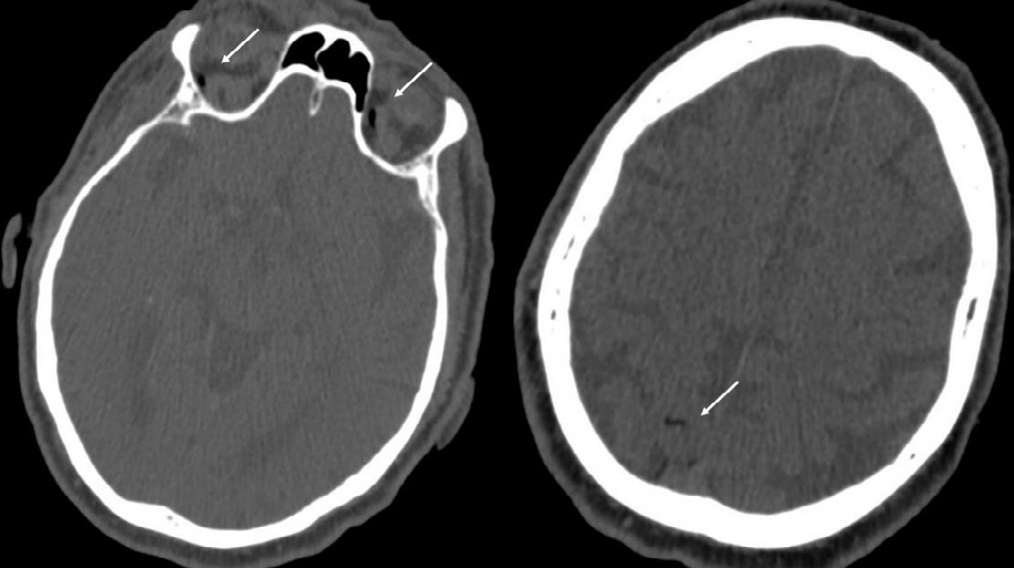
Acute head CT. Revealing air in the ophthalmic arteries on both sides and air in cortical vessels in the right parietal region. There are no signs of ischaemia at this time.
